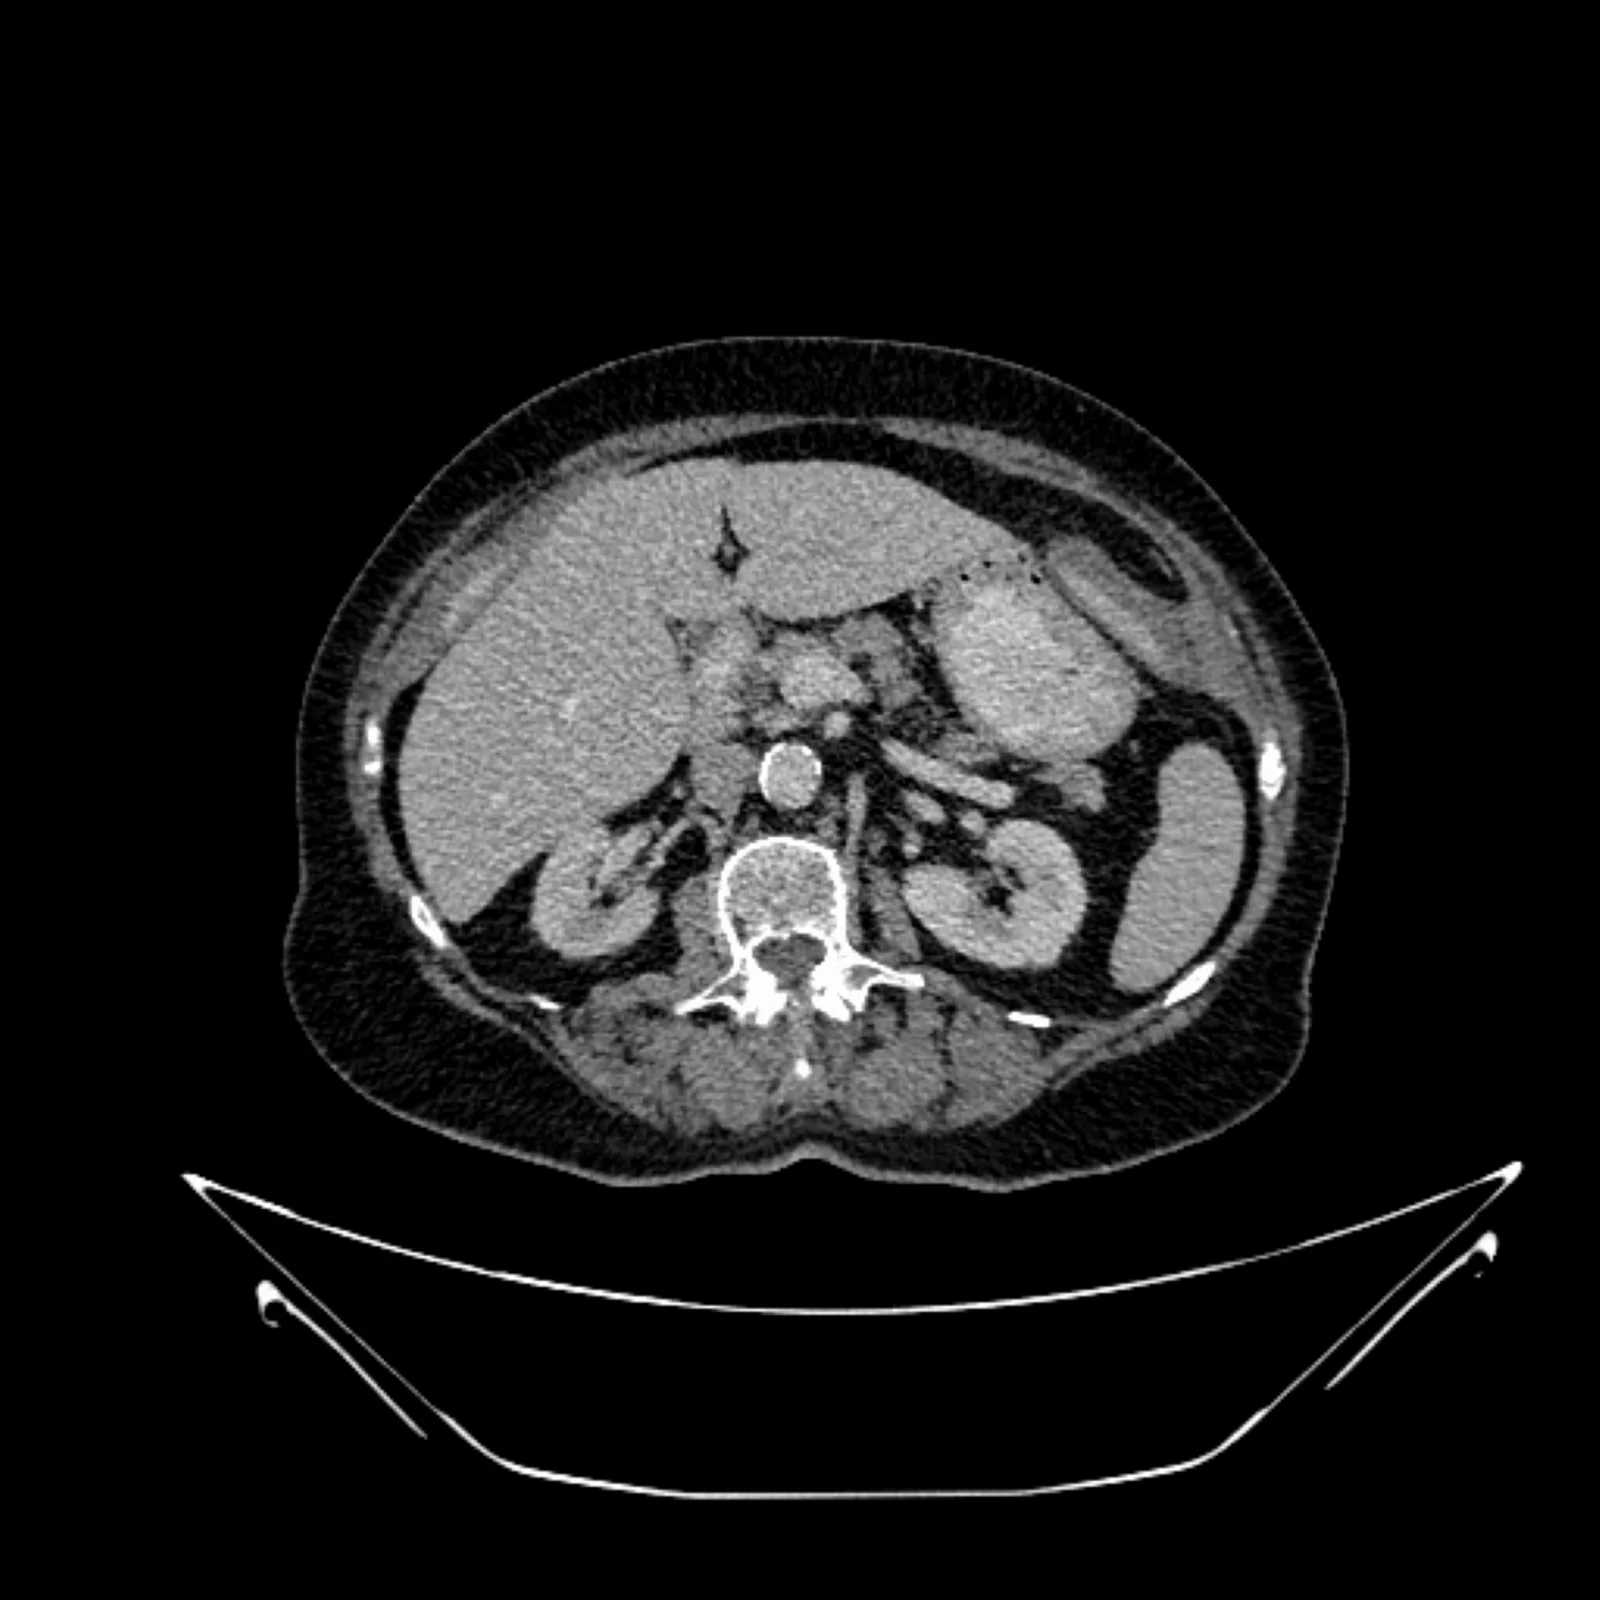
Cross-sectional CT image of the abdomen and pelvis showing a normal liver and pancreas, with no evidence of metastatic disease.
